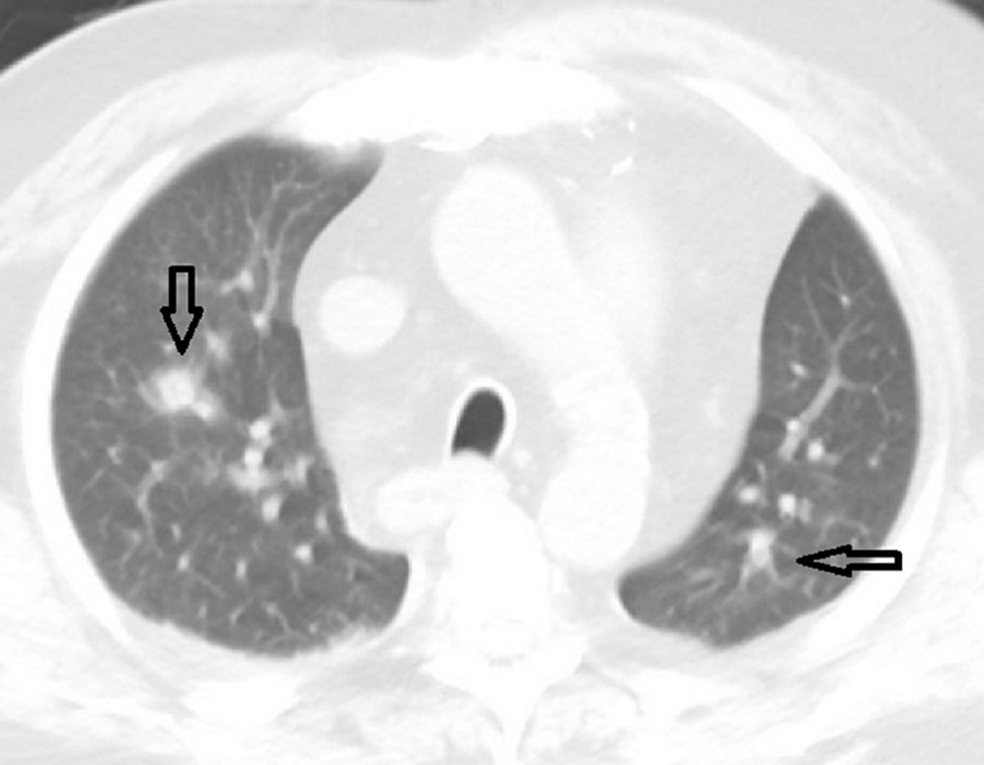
CT of the chest showing nodular multifocal consolidation bilaterally. CT: computerized tomography
